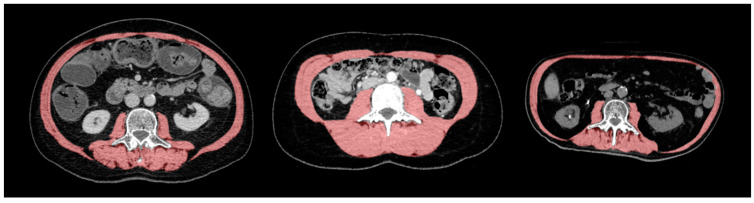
Skeletal muscle area morphology. Computed tomography scans show different morphotypes of skeletal muscle area (SMA) at the third lumbar vertebra (L3) level. This region contains the psoas muscle, paraspinal muscles (erector spinae, quadratus lumborum), and abdominal wall muscles (transversus abdominus, external and internal obliques, rectus abdominus).
